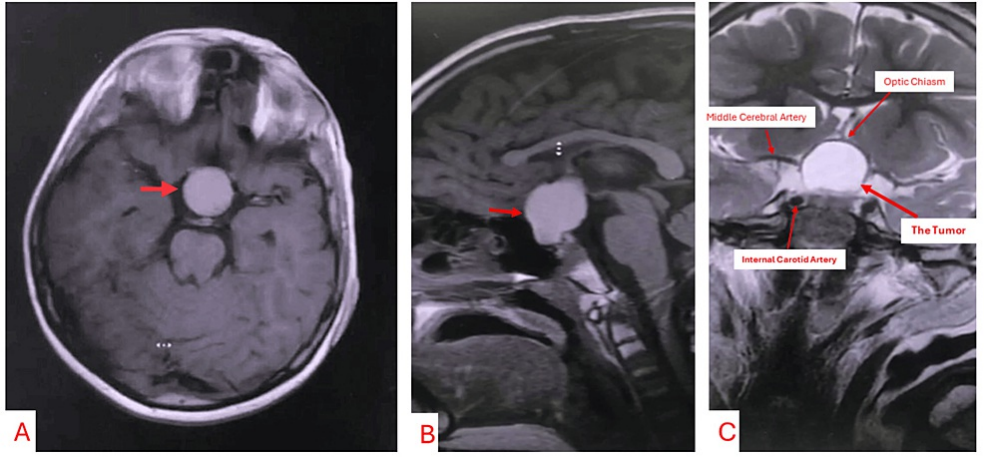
1a. T1 MRI of the brain in the axial plane at the level of rostral pons. The arrow points to the cystic, hyperintense part of the tumor. Hyperintensity reflects proteinaceous fluid. 1b. T1 MRI of the brain in the sagittal plane. The arrow points to the pear-shaped tumor, which is in a suprasellar-extraventricular location. No imaging signs of hydrocephalus. 1c. T2 MRI of the brain in the coronal plane. Significant distension of optic chiasm by the tumor. The chiasm is compressed from below and visible as a thin line over the superior surface of the tumor.
